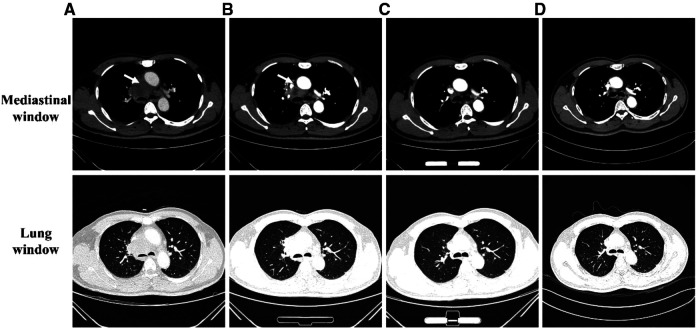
The dynamic changes in enhanced chest CT images at different time points. Mediastinal (top) and lung window (bottom). (A) At admission, Chest CT showed a mediastinal mass measuring 7.76 × 4.55 cm (arrow). (B) During discharge, Chest CT showed a decrease in the size of mediastinal mass to 5.53 × 4.51 cm (arrow). (C) After six months of treatment, Chest CT showed obvious absorbance of the mediastinal lesions. (D) One and a half years follow-up Chest CT showed obvious absorbance of the mediastinal lesions.
